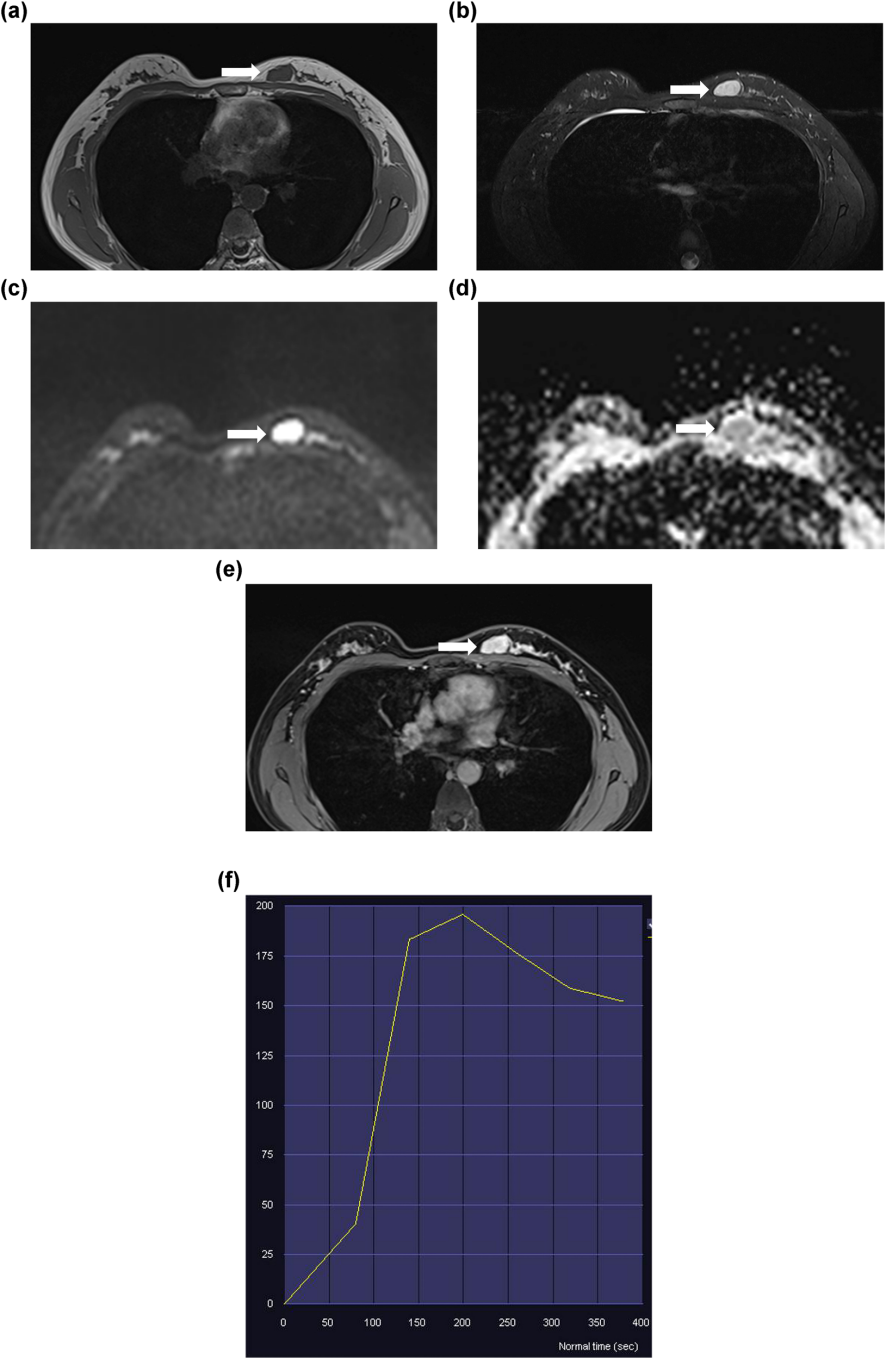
MRI of lesions of the left breast (arrows). (a) Lesions with Low signal intensity on T1-weighted images. (b) High signal intensity on T2-weighted images. (c and d) High apparent diffusion coefficient value. (e and f) Dynamic contrast-enhanced imaging with time signal intensity curves for washout pattern.
